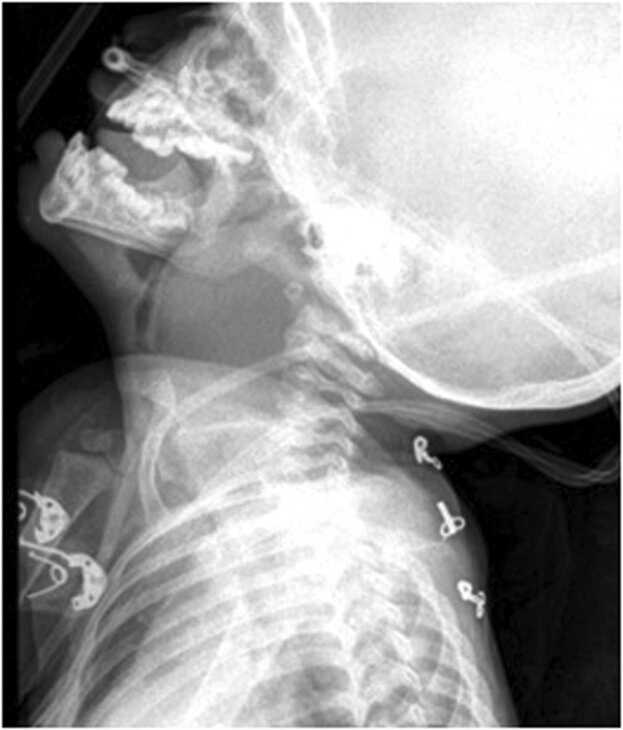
Plain lateral neck x-ray-reveals widening of soft tissue with anterior airway displacement. At the level of C2- 40 mm and at C6- 35 mm soft tissue widening is noted with extension of soft tissue shadow to the mid thoracic level.
